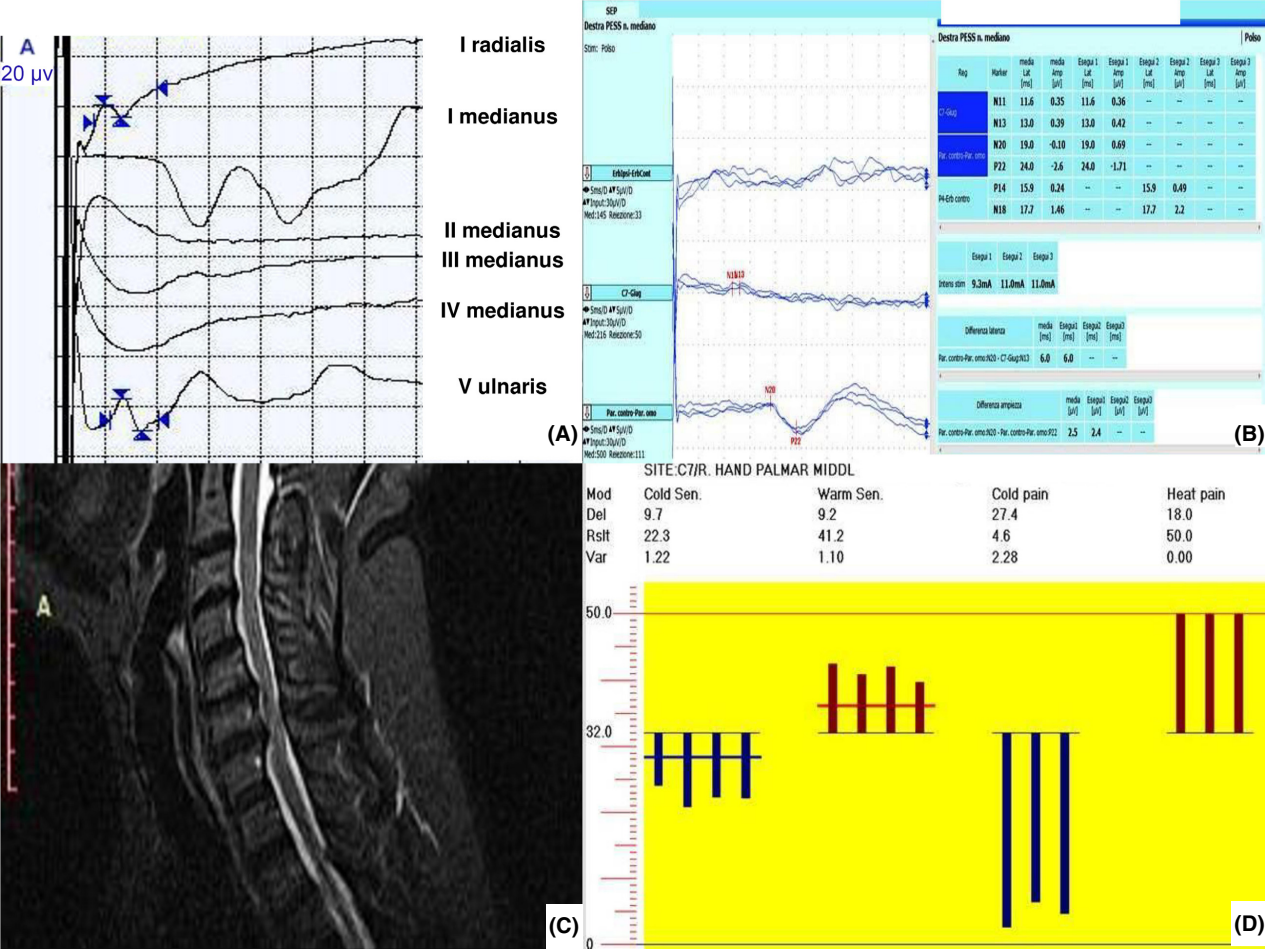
Imaging and neurophysiological tests. (A) Nerve conduction velocities; (B) median nerve somatosensory‐evoked potentials; (C) cervical MRI; (D) quantitative sensory testing.
